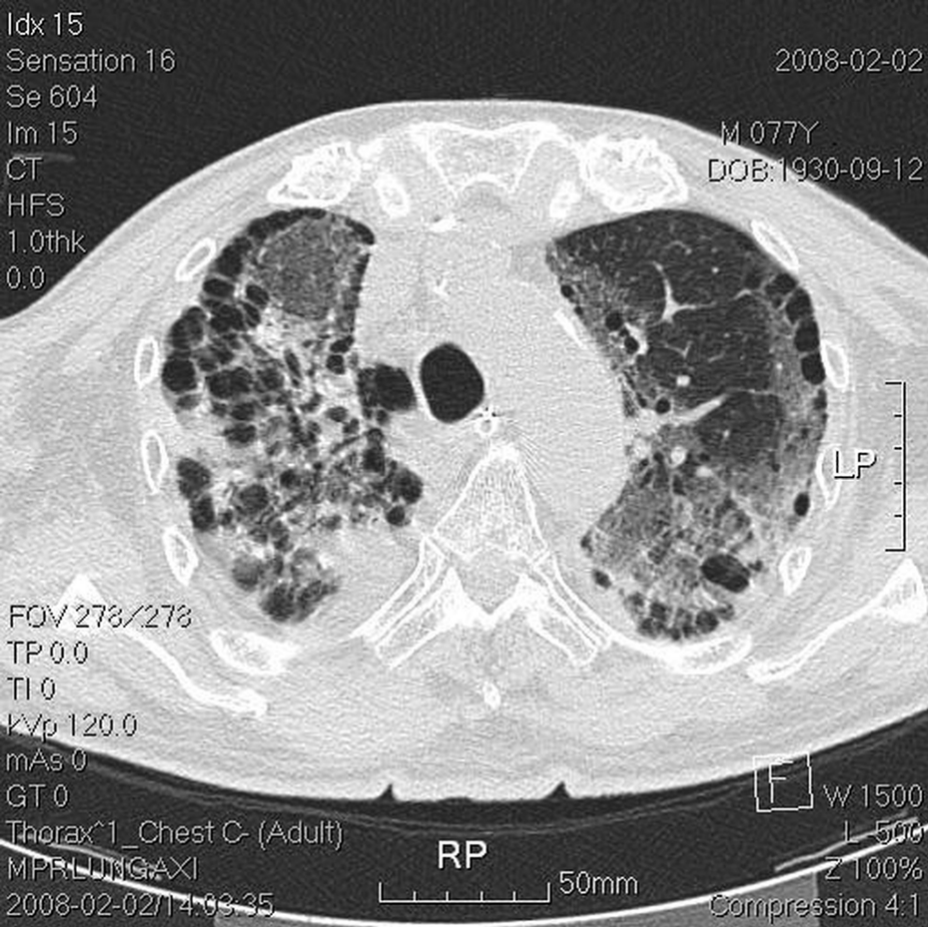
Transverse view of upper lung field with tumor located in the right upper lung apex. Chest computed tomography (CT) done post intubation shows ground-glass opacities, blebs confined to the right upper lung apex, and diffuse ground-glass attenuation, blebs in the marginal areas, airspace consolidation, and fibrosis in the bilateral upper and lower lung fields.
